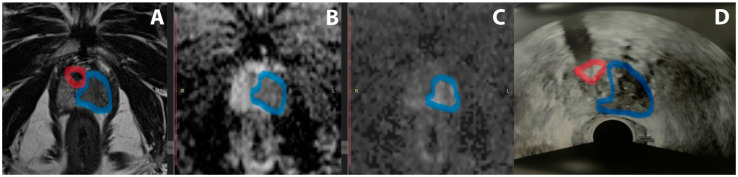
Example of PI-RADS 5 lesion. T2 (A), ADC (B), DWI (C), and mpMRI sequences and TRUS (D). The suspicious lesion is highlighted in blue, and a calcification used as a reference point for performing the tPBx is highlighted in red. mpMRI: multiparametric magnetic resonance imaging; PI-RADS: Prostate Imaging–Reporting and Data System; tPBx: targeted prostate biopsy; TRUS: transrectal ultrasound.
